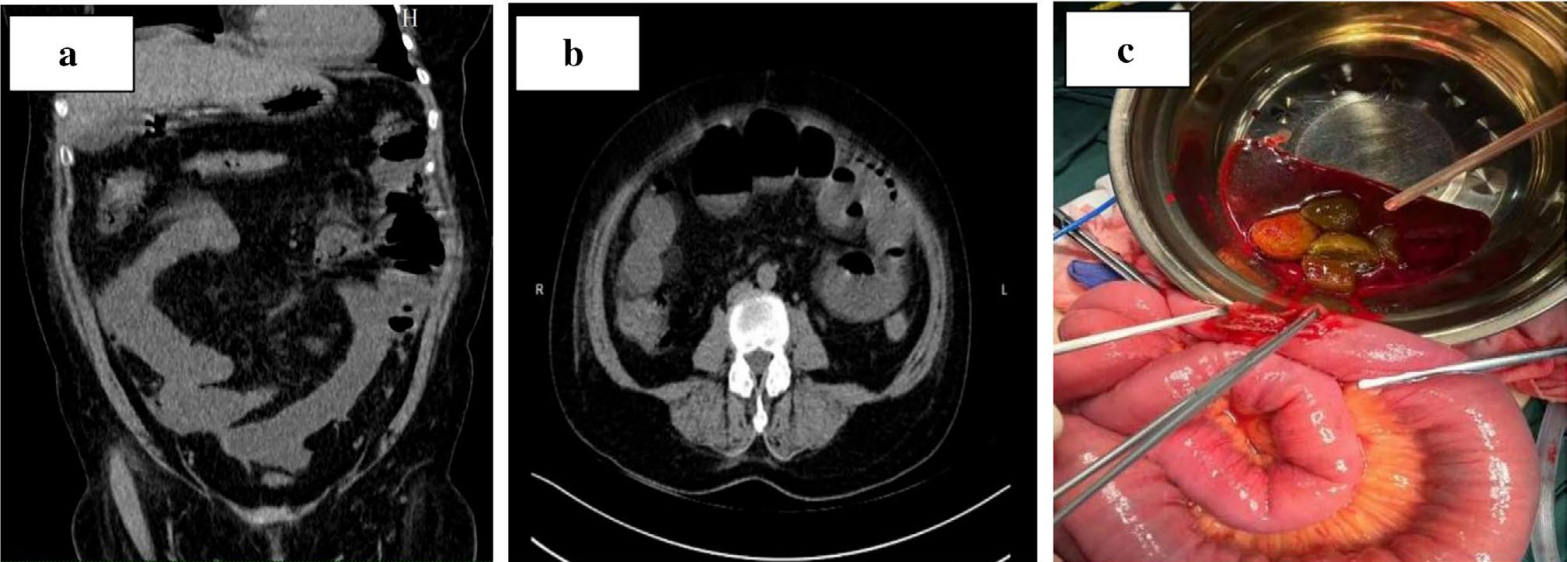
(a) Computed tomography. Small bowel obstruction with suspected ileocecal wall thickening (coronal view). (b) Computed tomography. Small bowel obstruction with suspected ileocecal wall thickening (axial view). (c) During the operation, exploratory laparotomy and small bowel incisions were performed to remove fecal stones.
